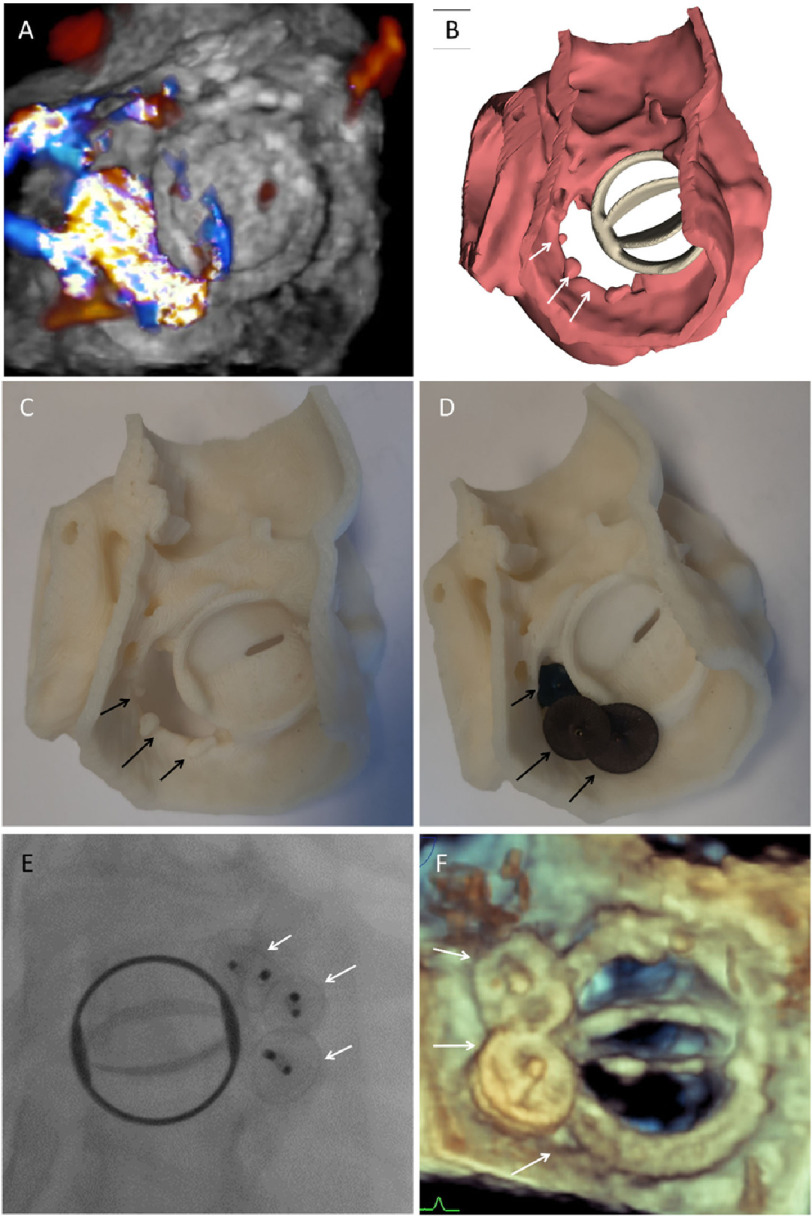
The second case. A. 3D TEE showing the defect at 6–10 o’clock. B. CT Segmentation showing the defect. C. 3D model of the defect. D. Bench test using three AVP II. E. Percutaneous closure using planned plugs. F. 3D TEE showing well seated plugs.
